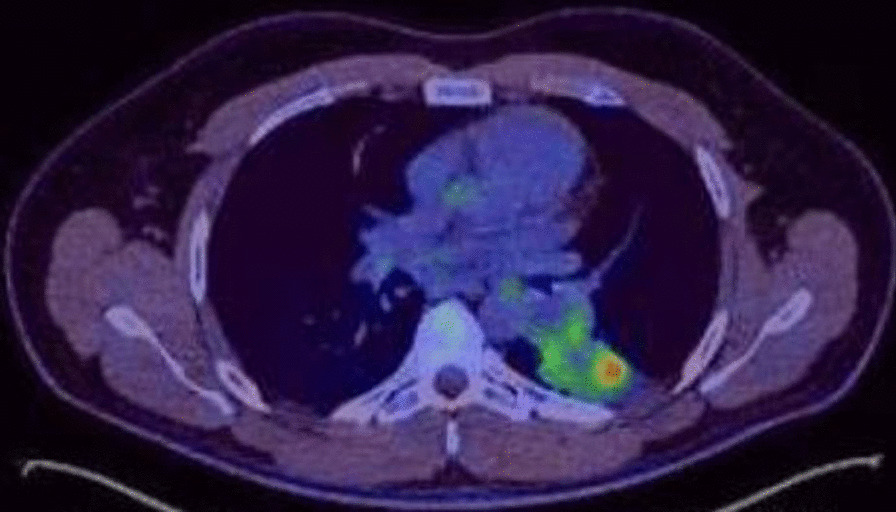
Pre-operative PET-CT slice to correlate findings from Fig. 2. Within this consolidation, there is impression of a rounded abnormality on the PET component showing nodular peripheral activity (SUV Max 8.7) with central inactivity. This measures approximately 3.4 cm × 3.3 cm. The remainder of the consolidation shows no significant activity
